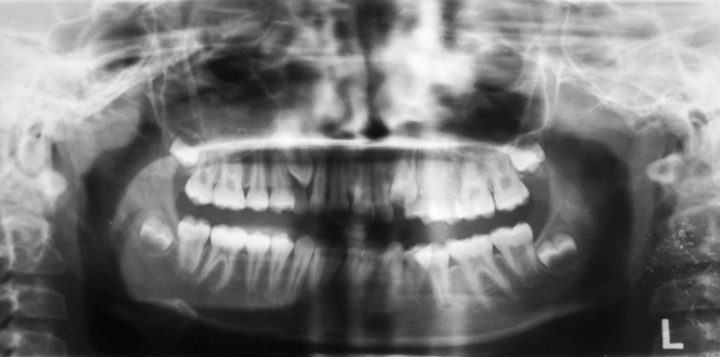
Orthopantomograph for orthodontic diagnostic purposes
